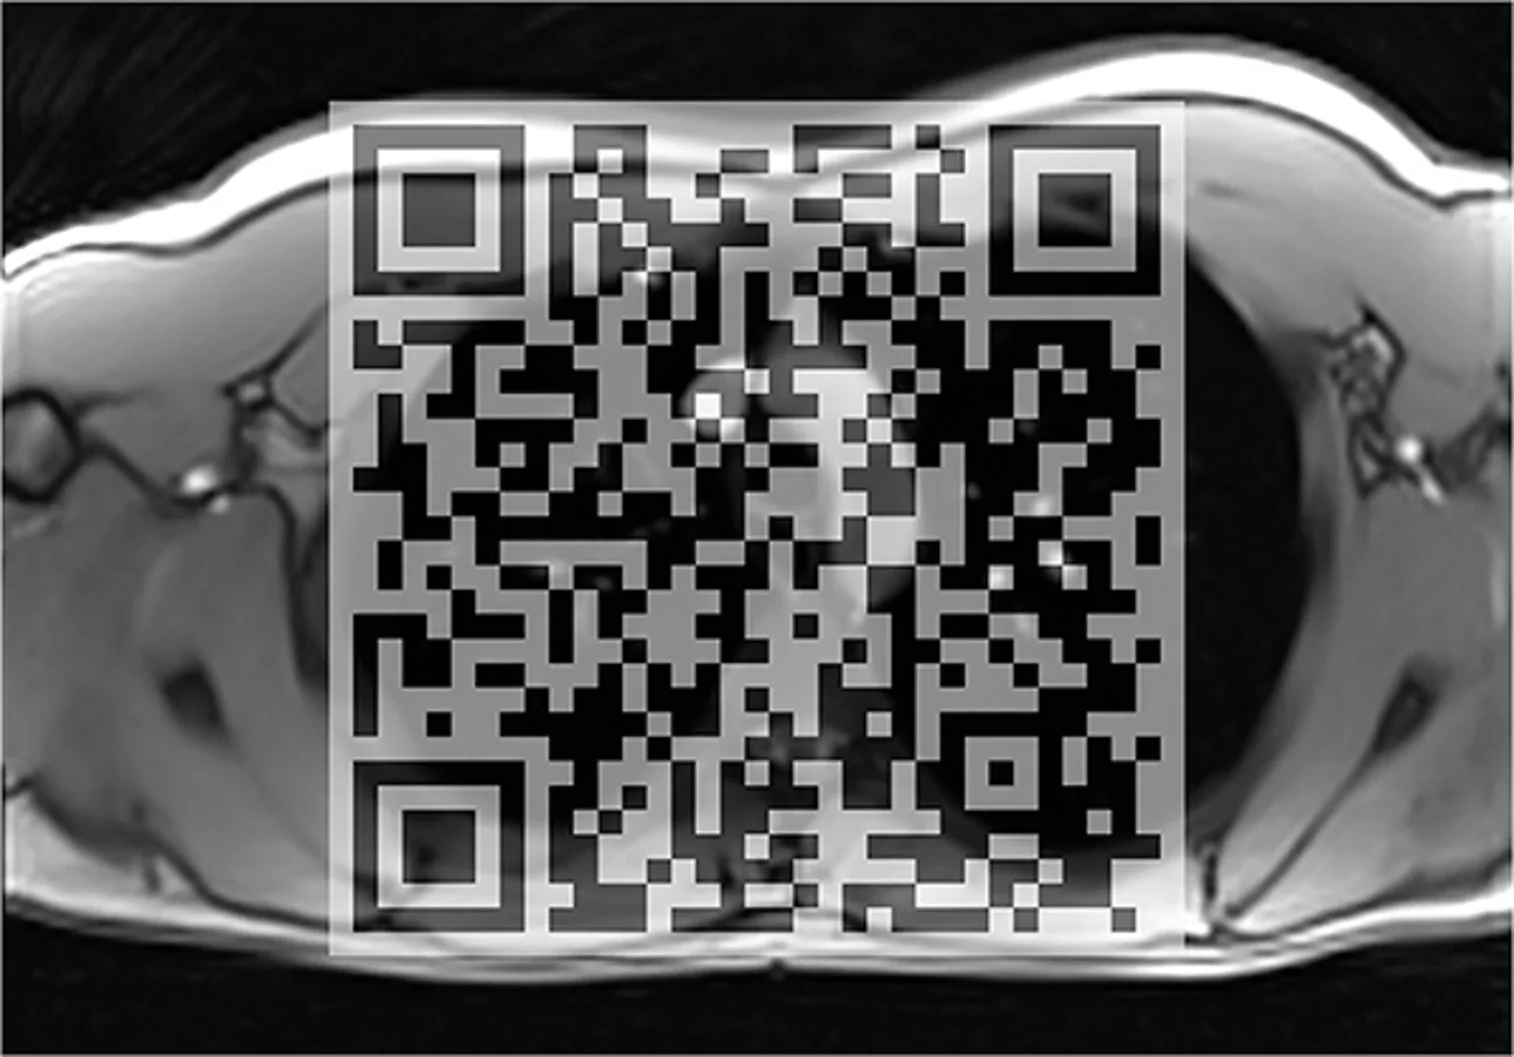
Real-time MRI of a 24-year-old patient following neonatal repair of esophageal atresia. In the axial plane, reduced motion of the right hemithorax at the level of a rib fusion (Ribs 4 and 5) is clearly visible. (https://pedz.de/supplementals/ea-adult/video1.mp4).
